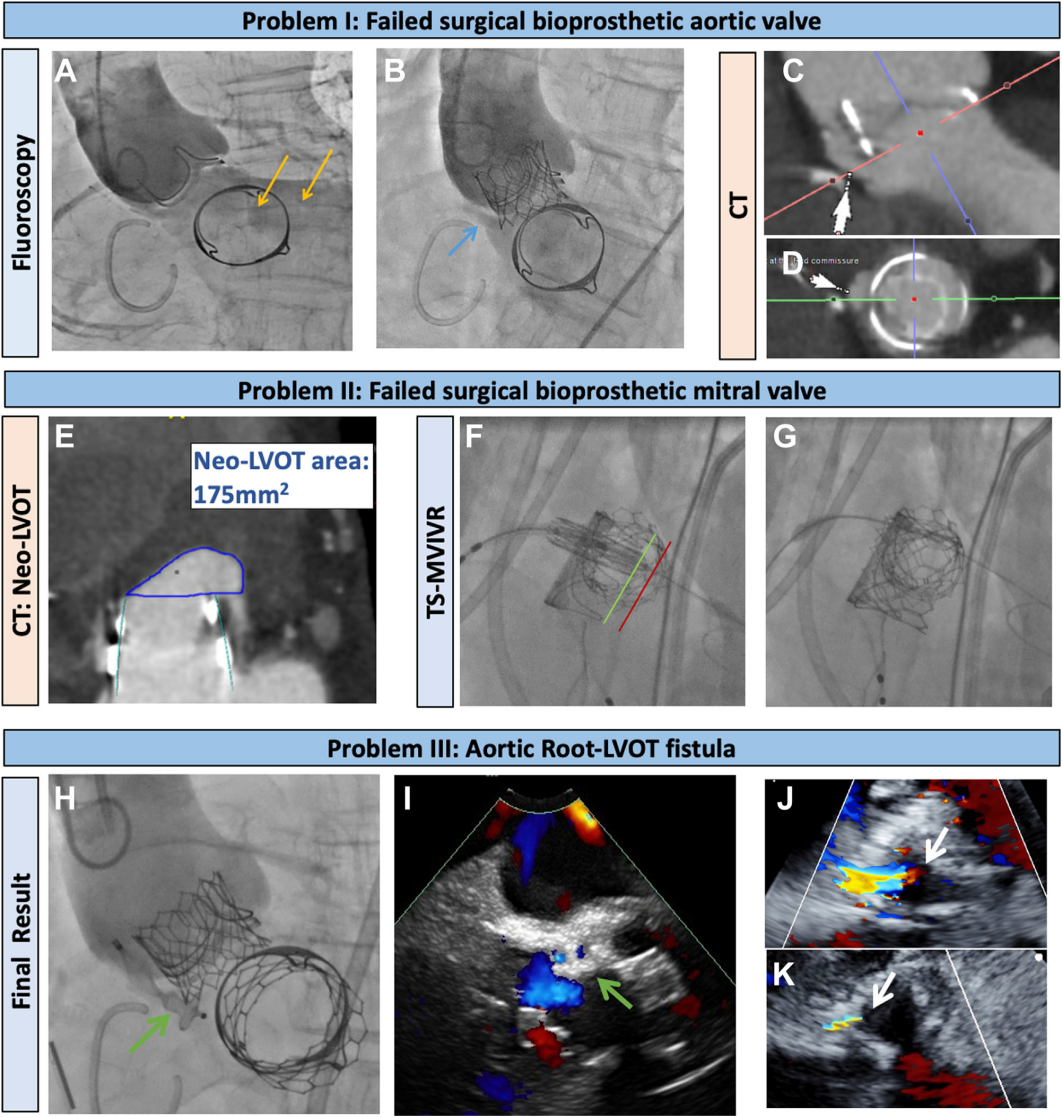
Managing multivalvular failure and hidden aortic root–LVOT fistula. (A) Aortic root angiogram pre-VIV indicates severe regurgitation (yellow arrows). (B) Post-VIV demasks communication (blue arrow) between aortic root and LV, which was obscured by dominant transvalvular regurgitation. (C, D) Pre VIV computed tomography (CT) revealed dehiscence at the level of sewing ring of bioprosthetic aortic valve at non- and right coronary commissure (white arrows). (E) High anticipated risk of Neo-Left-Ventricular-Outflow-Tract (Neo-LVOT) obstruction on CT. (F, G) Ventricular portion of THV (green line) positioned in line with the stent posts of surgical bioprosthesis to minimize risk of Neo-LVOT obstruction with a good final result. (H, I) Final aortic root angiogram after paravalvular leak closure under fluoroscopic and ICE guidance showing good position of the device (green arrows) with significant reduction of paravalvular regurgitation. (J, K) TTE pre- and post- paravalvular leak closure with a 10 × 5 mm Amplatzer Valvular Plug III device confirming significant reduction in paravalvular regurgitation (white arrows). ICE, intracardiac echocardiogram; LV, left ventricle; THV, transcatheter heart valve; TS-MVIVR, transseptal mitral valve in valve replacement; TTE, transthoracic echocardiogram; VIV, valve in valve.
